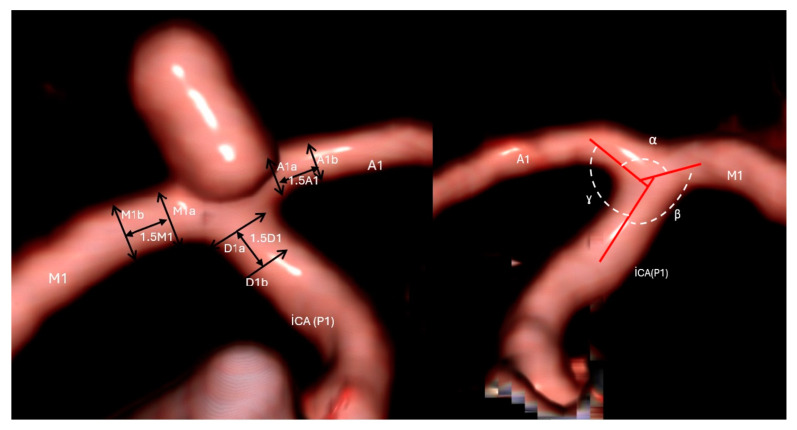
Three-dimensional CTA model of the ICA bifurcation depicting morphological variables of the surrounding vasculature. M1 = middle cerebral artery proximal segment, A1 = diameter of anterior cerebral artery proximal segment.
